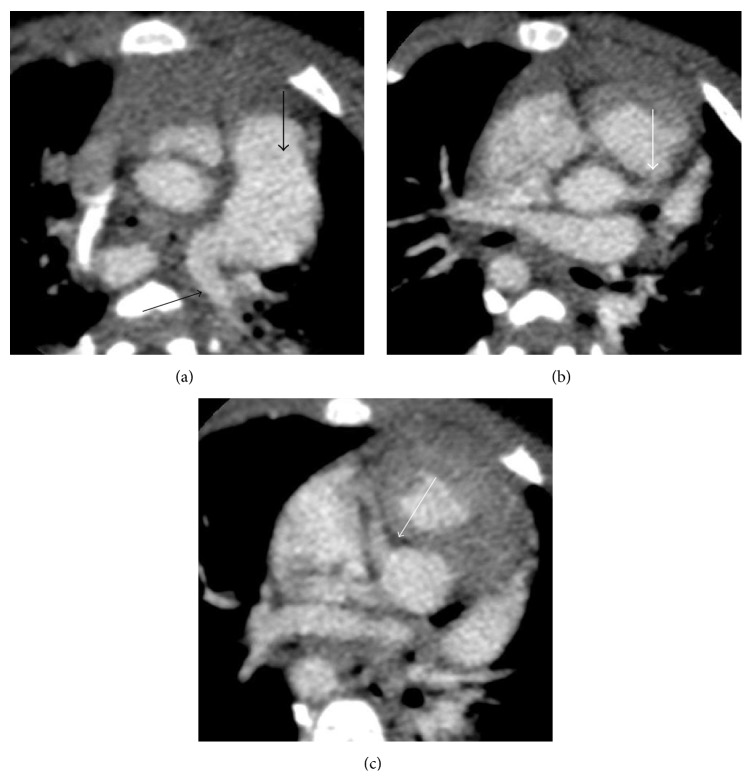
Multiple slices from a postcontrast CTA of the chest from superior to inferior demonstrate enlargement of the pulmonary artery (thick black arrow) and crisscross pattern of the pulmonary arteries (thin black arrow). There is dilatation of the origins of the left coronary artery (thick white arrow) and right coronary artery (thin white arrow).
